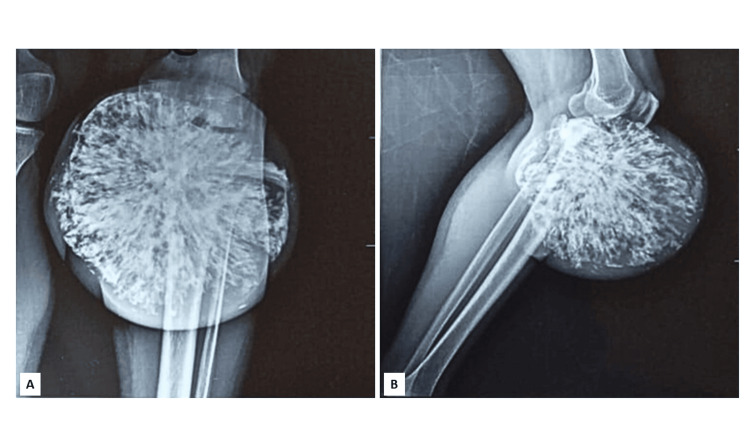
X-ray showing antero-posterior (A) and lateral (B) views of the left knee with a large radiolucent area with radiating calcific densities extending from the center (typical sunburst or sunray appearance)
